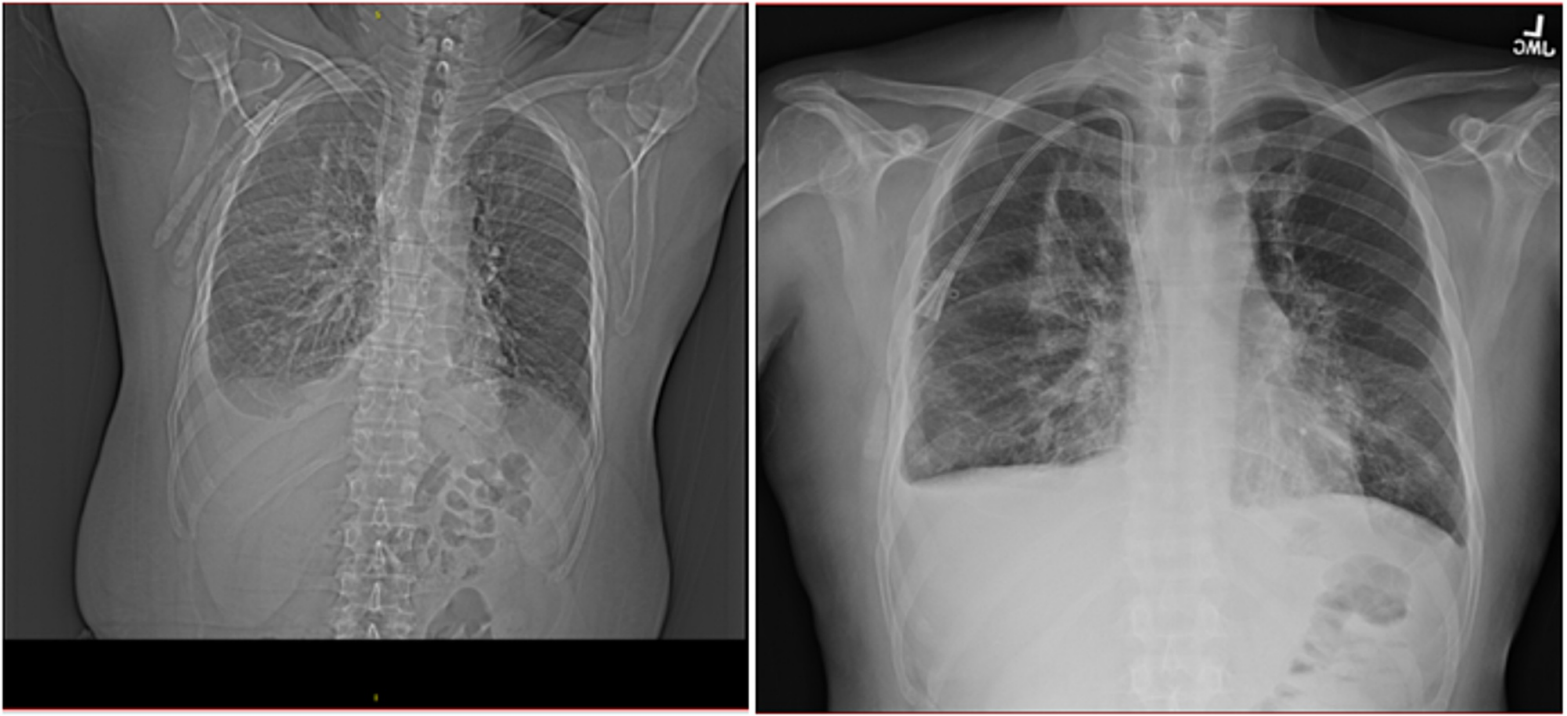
Chest CT scan: day 5 versus day 9.
